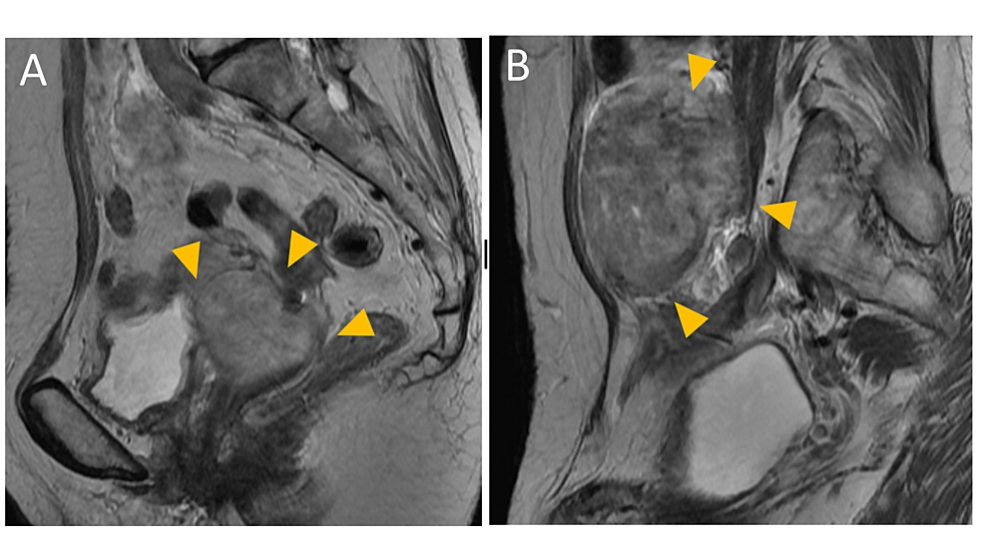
T2-weighted sagittal magnetic resonance imaging (A) Pelvic mass above the vaginal cuff (arrowheads). (B) Abdominal mass under the umbilicus (arrowheads). On the right side of the vaginal cuff, a nodule with a long diameter of 55 mm was found, with slight high intensity and relatively uniform enhancement on T2-weighted images. On the left side of the pelvis, there was a well-demarcated, solid mass lesion with a long axis of about 10 cm, showing slight high intensity on T2-weighted images. The contrast enhancement was similar to that of the vaginal cuff nodule, and on diffusion-coordinated imaging, both masses were hyperintense, but without a clear tendency to invade surrounding structures. Enlargement of residual uterine myomas and metastatic myomas were suspected.
